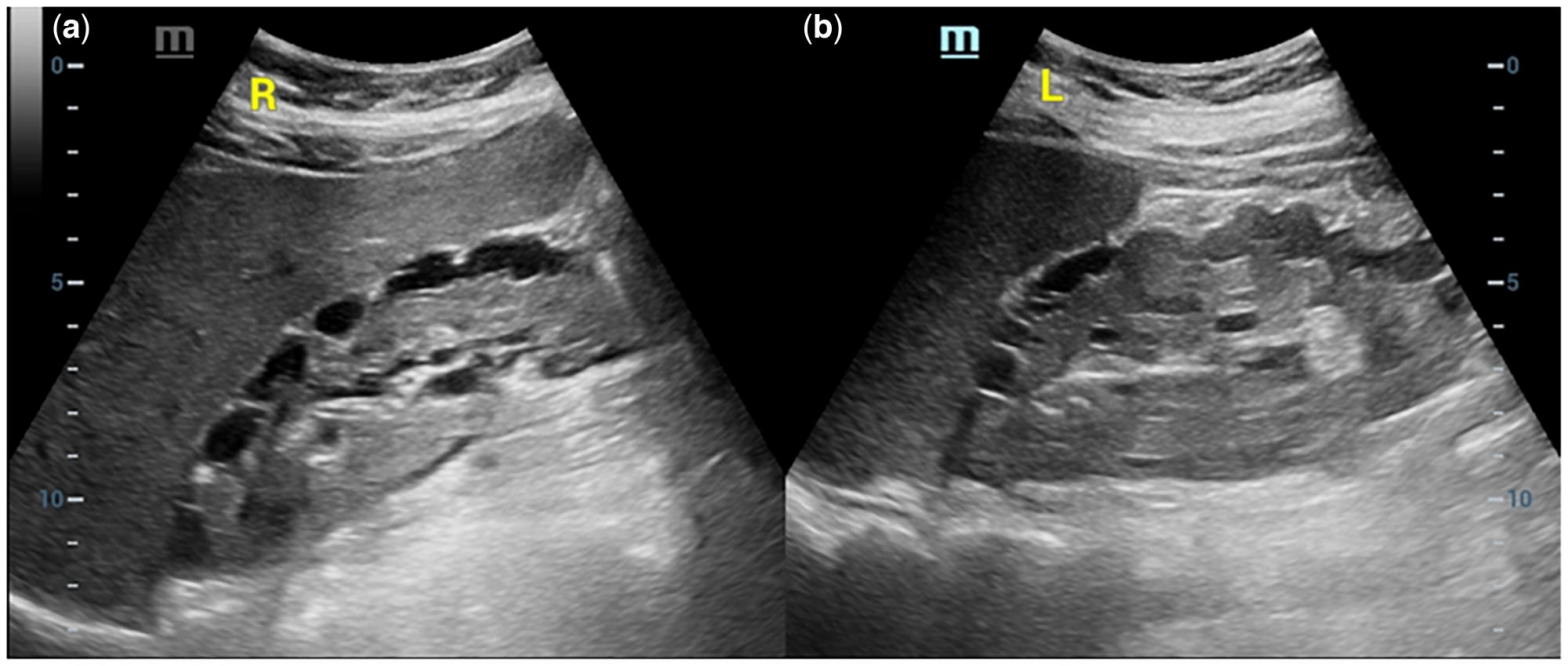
Ultrasonography of the right (A) and left kidney (B) in the longitudinal axis demonstrates bilateral perirenal and intrarenal cystic lesions.
